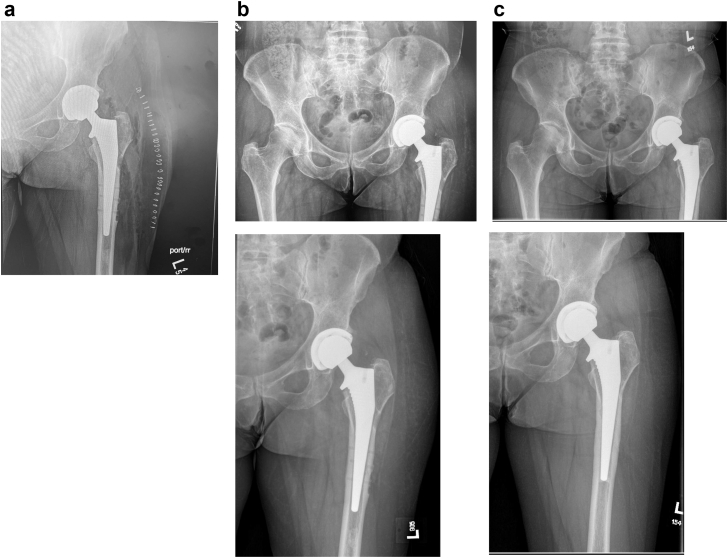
(a) Immediate postoperative radiograph; (b) 1-month postoperative radiograph; and (c) 9-month postoperative radiograph.
